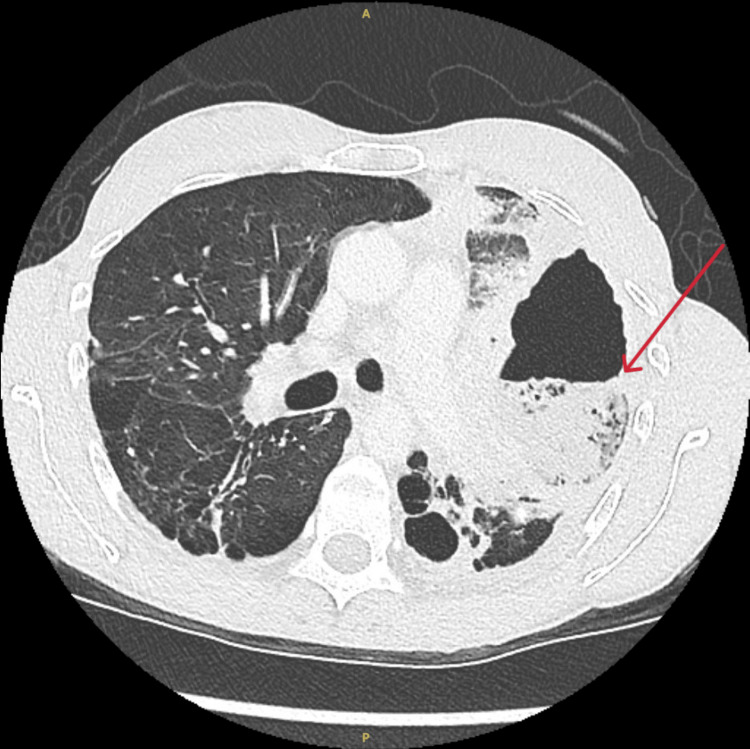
Computed tomography of the chest shows an abscess in the left upper lobe containing an air-fluid level.
